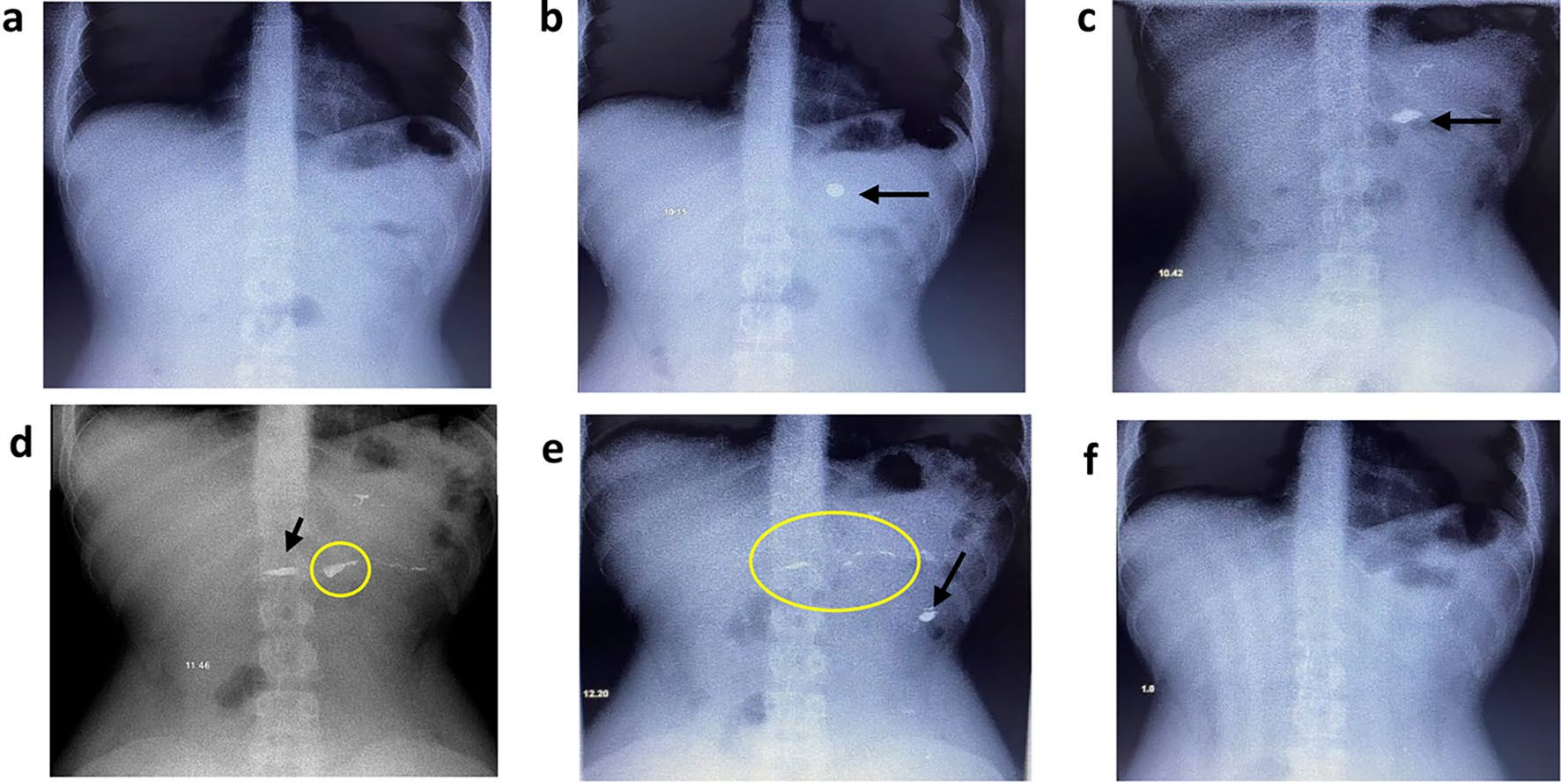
Radiographic images of the location of the contrast metal tablet (F5) in the stomach of a mature human: (a) Pre swallowing tablet. (b) Post swallowing tablet. (c) After 30 min. (d) After 1.5 h. (e) After 2.5 h. (f) After 3 h.
